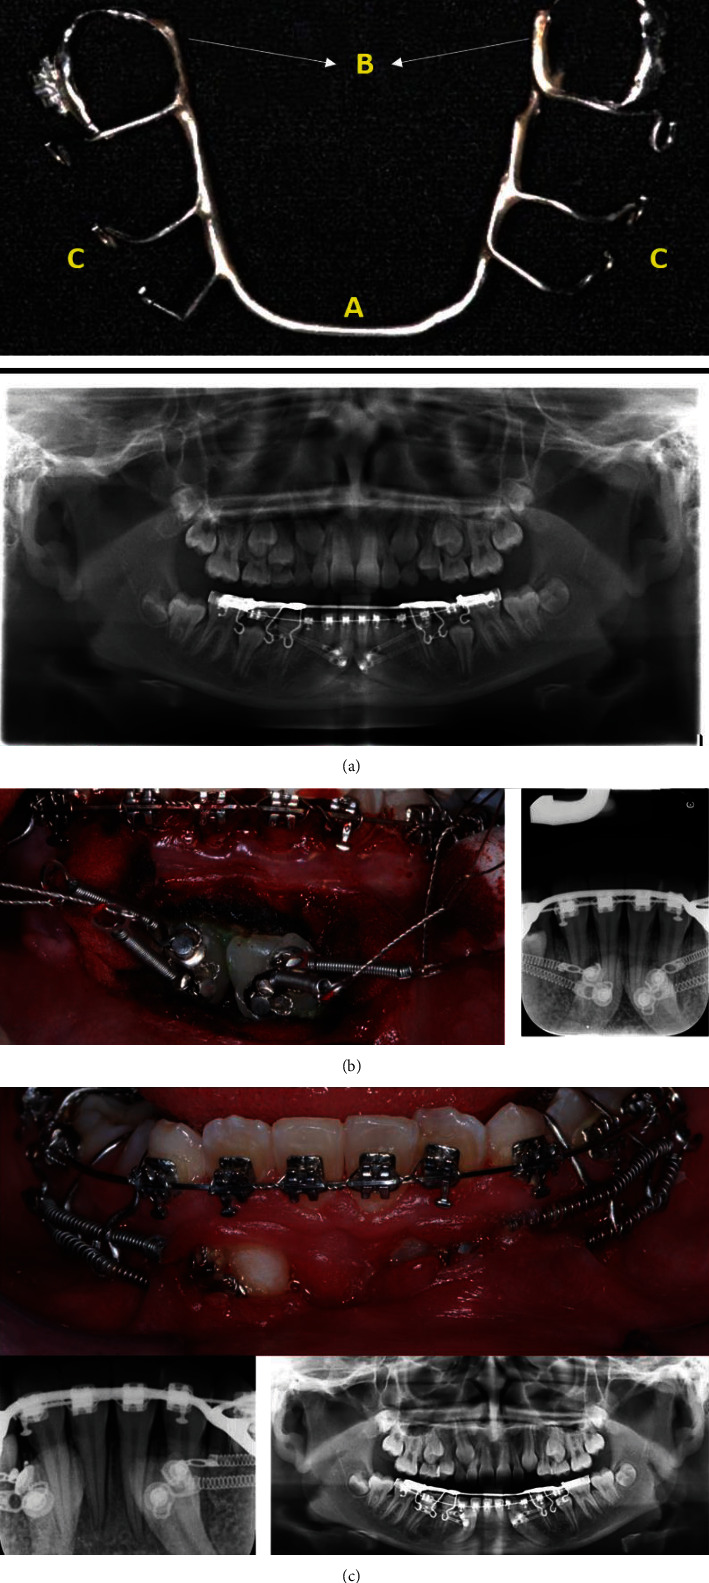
(a) Fixed device for temporary anchorage. (A) Lingual arch main support in 1.1 mm SS wire. (B) Prefabricated metal bands with lower first molar tubes. (C) Vertical hooks in 0.028″ SS wire, with bends towards the distal direction. (b) Trans-surgical radiographic and clinical records. (c) Three months of traction. Radiographic and clinical records.
